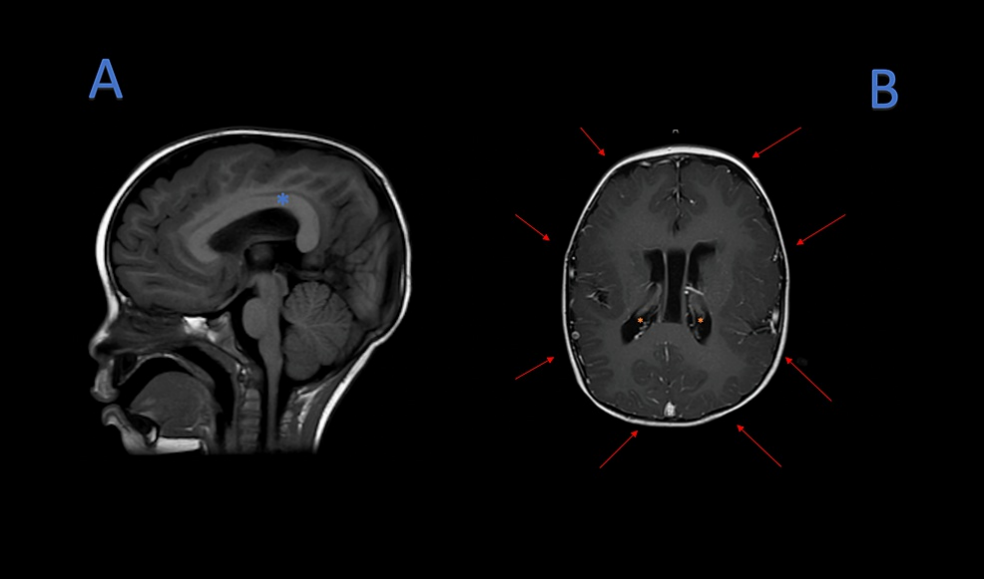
(A) MRI T1 sequence, shows thickening of the corpus callosum (blue asterix); (B) MRI T1 sequence, shows pachygyriam (red arrows), subependymal neuronal heterotopias (orange asterix). MRI: magnetic resonance imaging
